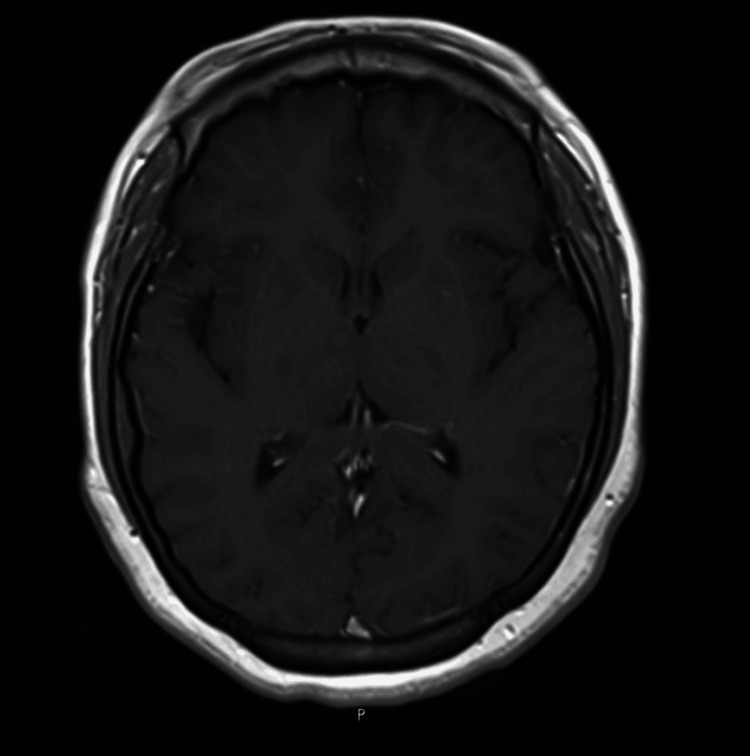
T1-weighted MRI showing normal lateral ventricles, basal nuclei, thalami, and normal-appearing white and gray matter. FLAIR MRI: Fluid-attenuated inversion recovery magnetic resonance imaging
